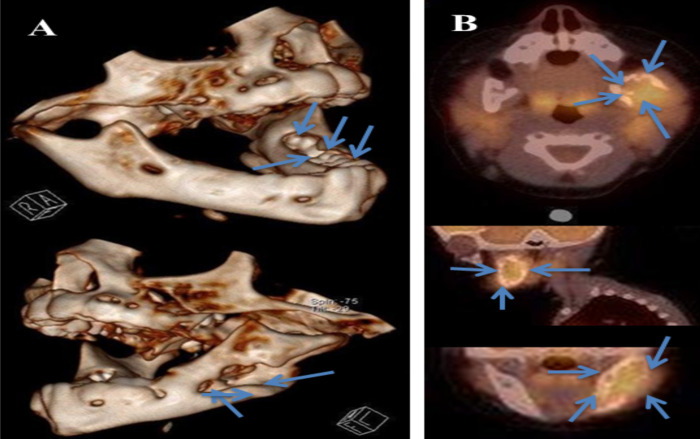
A. CT scan showed peri-apical osteolysis of several teeth on left mandible (arrows). The osteolytic lesion occupying the cortex of left hemimandible, chin spine, the body, ramus and the left mandibular condyle measured 4x2x3cm. This lesion demonstrated low enhancement with contrast media and seemed respecting the adjacent soft tissues. Slight pushback of fatty parapharyngeal space was seen, without important obliteration or densification. There was no mass effect on the level of oropharyngeal pathway or adenopathy. B. FDG PET/CT revealed an osteolytic lesion of left mandible (arrows).
